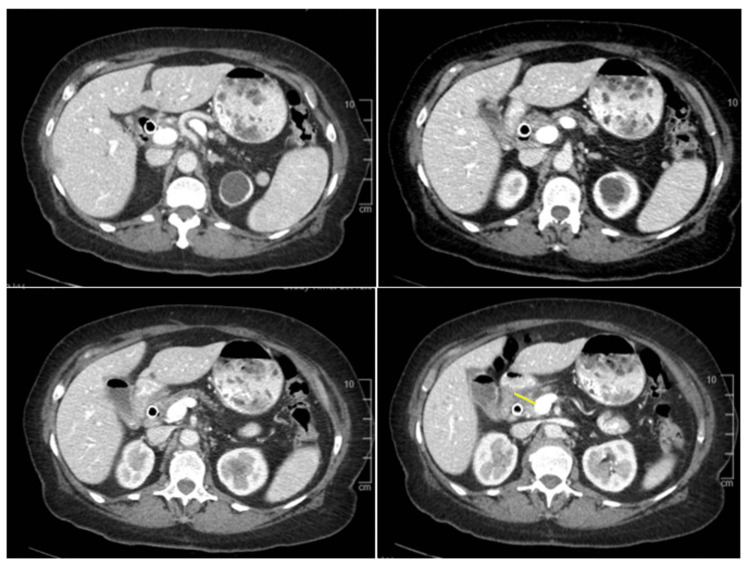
CT imaging of the pancreatic tumour at 3.8 months from diagnosis. The yellow bar indicates the longest tumour diameter CT: computed tomography
